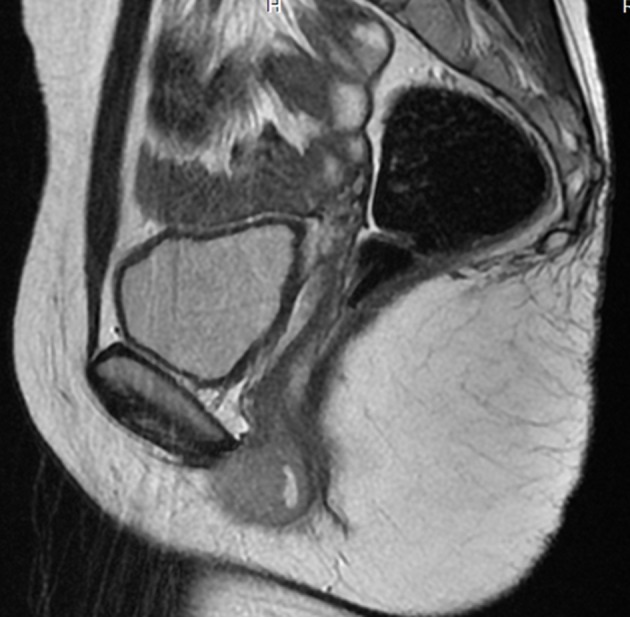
MRI T2WI SAG-lesion appears hyperintense.
